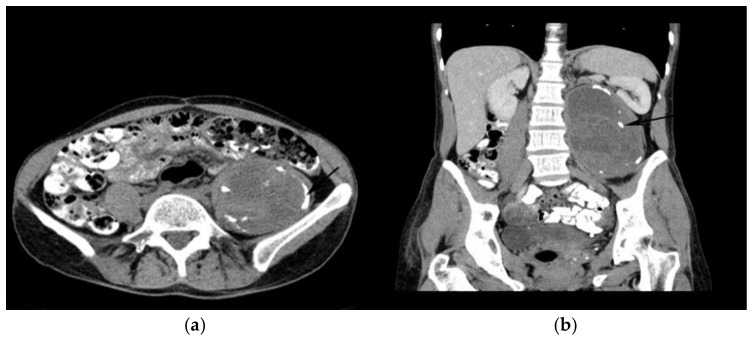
CT images in axial (a) and coronal (b) views show a distinct formation within the left psoas muscle.
